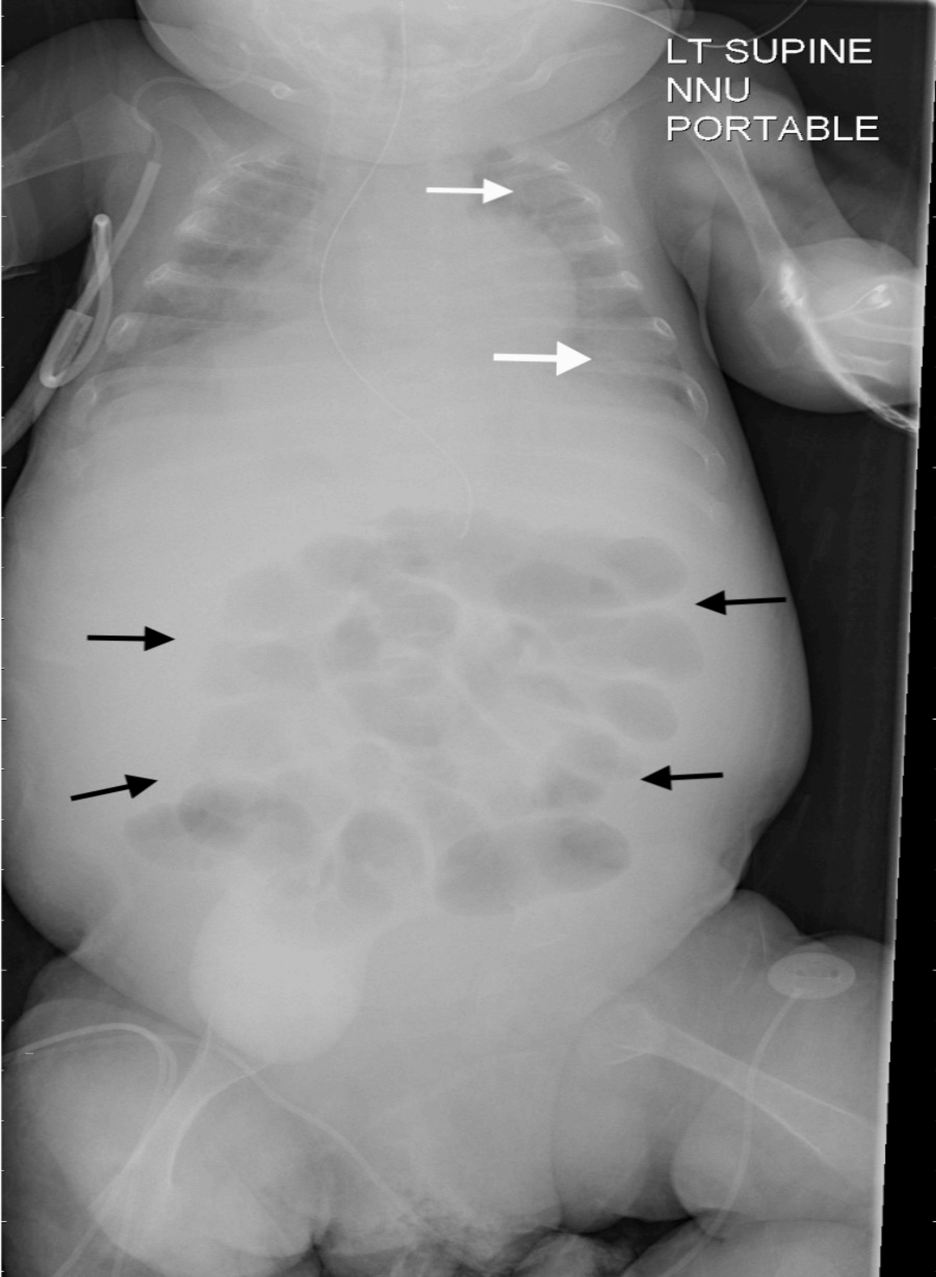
Chest and abdominal X-ray Chest and abdominal X-ray taken on day 81 of life, following respiratory deterioration. There is left upper and lower lobe consolidation (white arrows), as well as small lung volume bilaterally. Due to ascites, there is poor definition of the liver, and bowel loops are displaced to the centre of the abdomen (black arrows).
